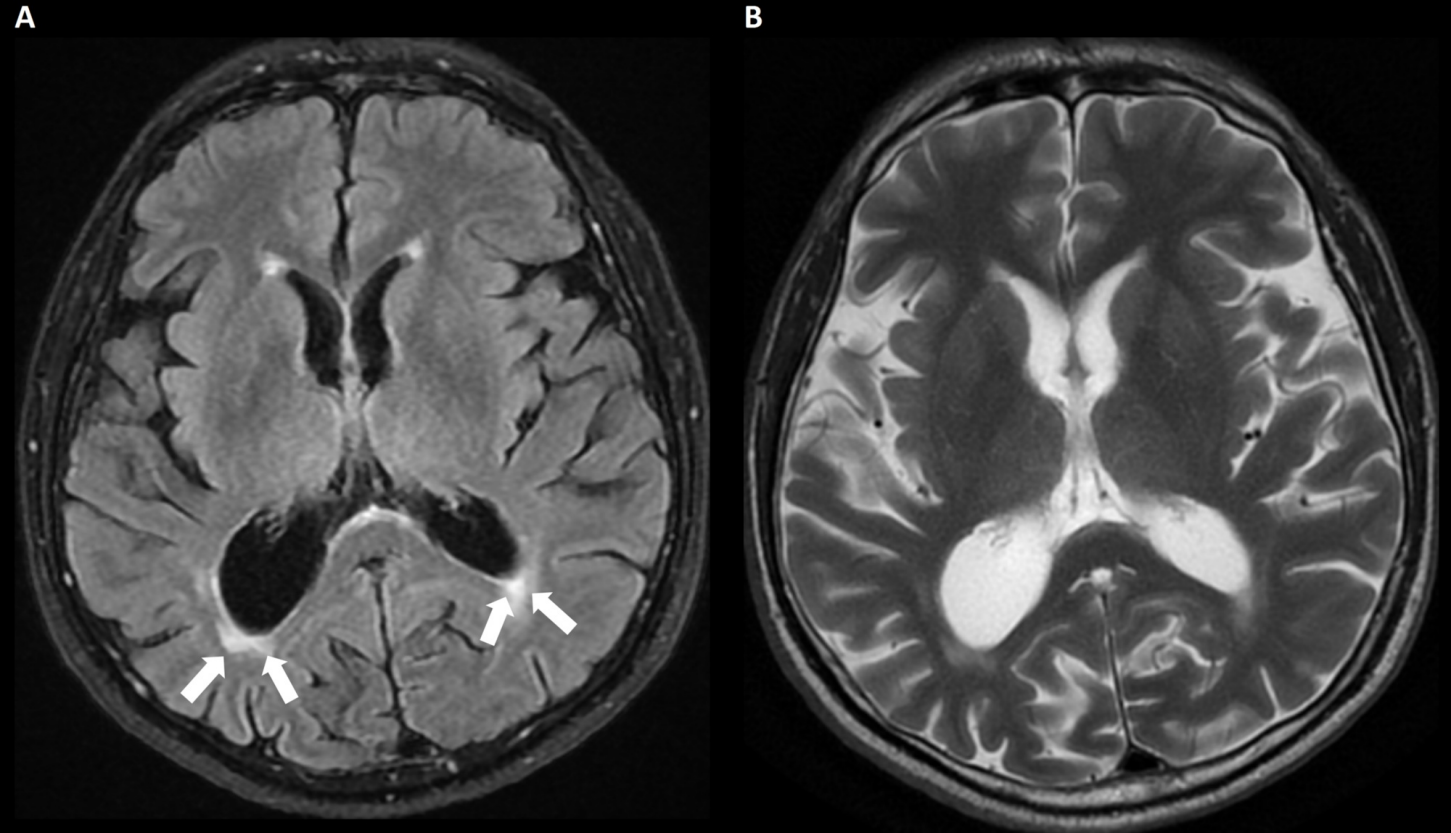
A three-month follow-up MRI. (A) FLAIR image. (B) Axial T2 image. Non-specific mild periventricular hyperintensities on FLAIR image (arrows) can be seen, with otherwise unremarkable features at the basal ganglia or hippocampal regions. FLAIR, fluid-attenuated inversion recovery
